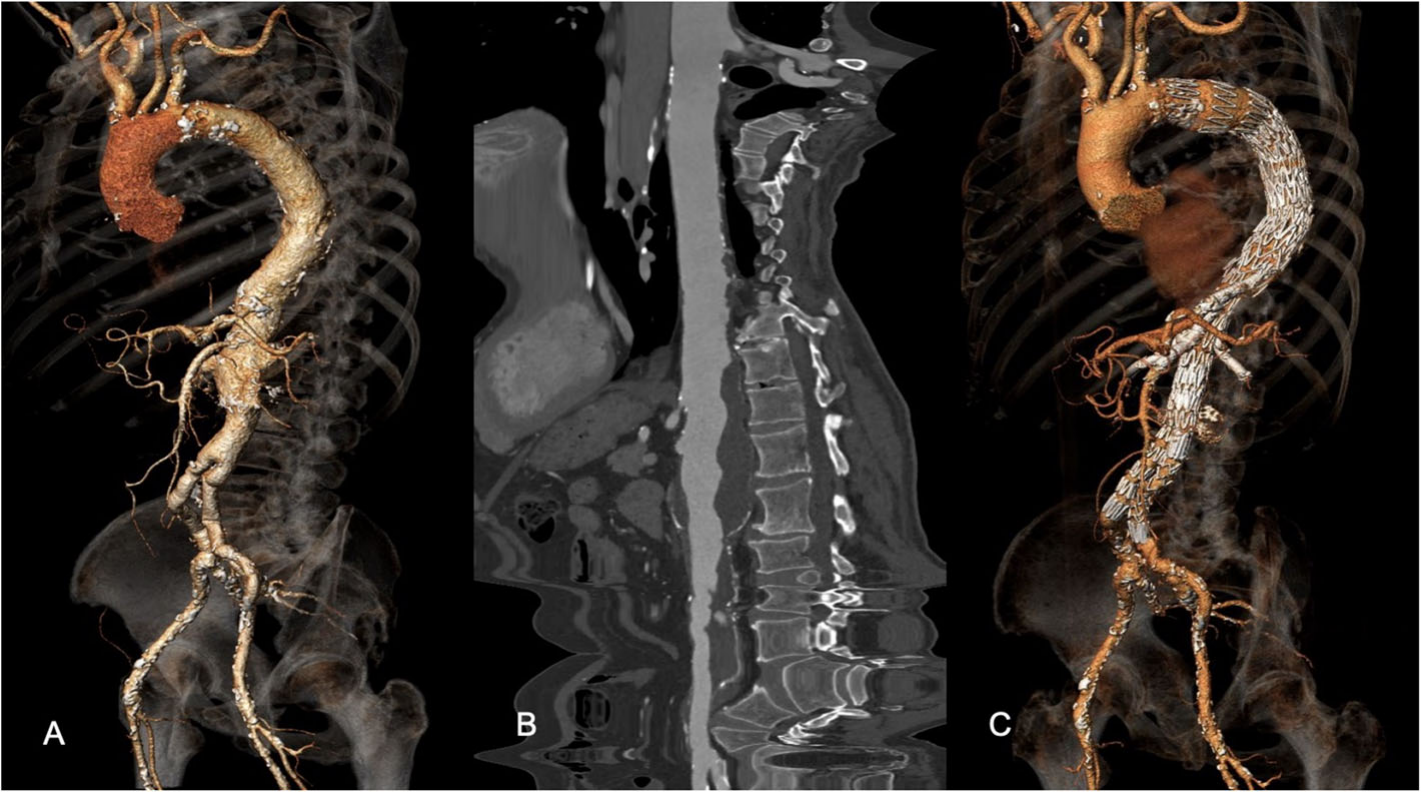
a, b Preoperative 3D volume rendering and vessel analysis of the CTA. c 3D volume rendering at 12 months showing exclusion of the TAAA and patency of the visceral vessels. Print color requested
